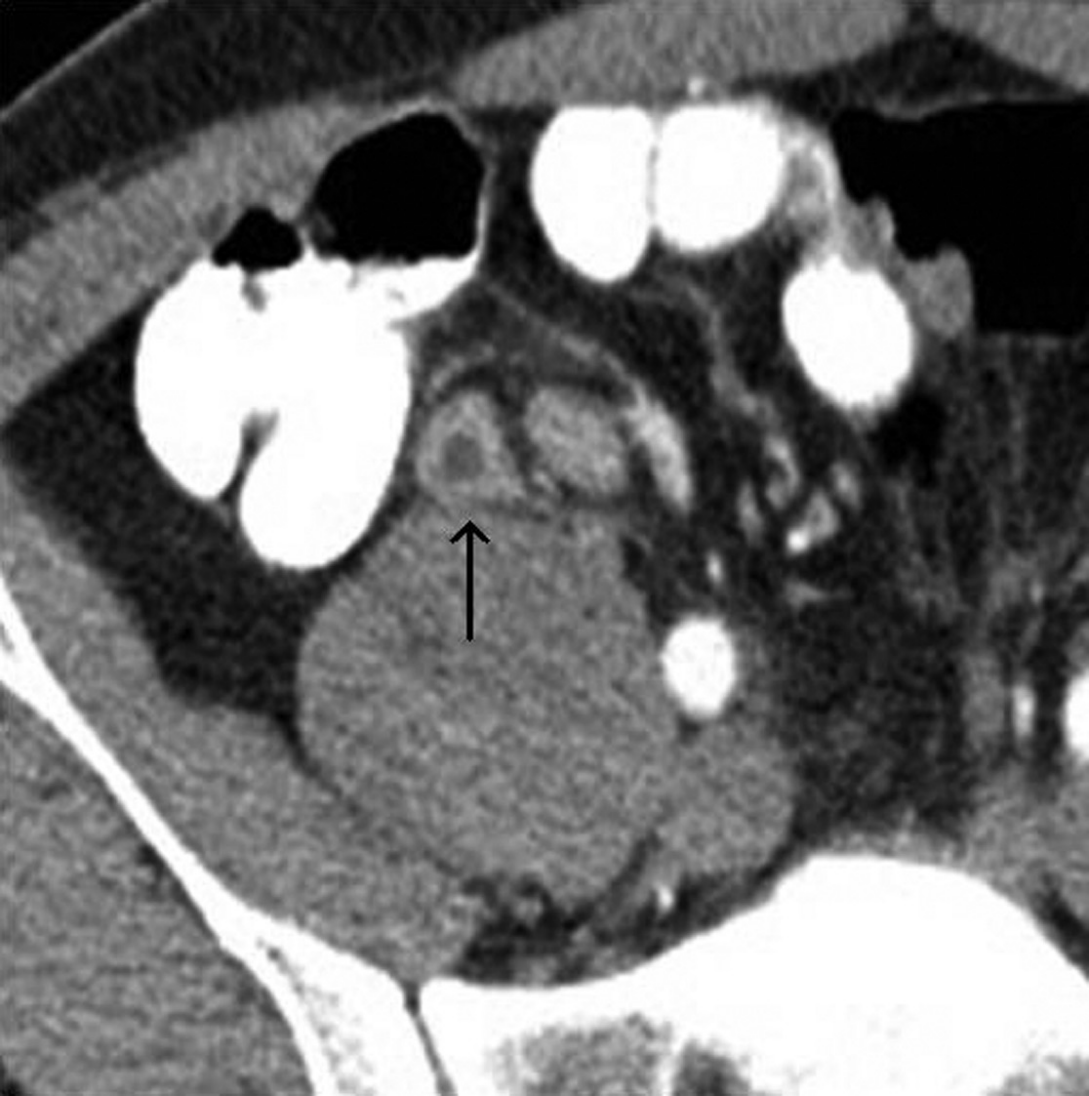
Axial CT scan shows an inflamed, thick-walled appendix with peri-appendiceal inflammatory stranding (arrow).
